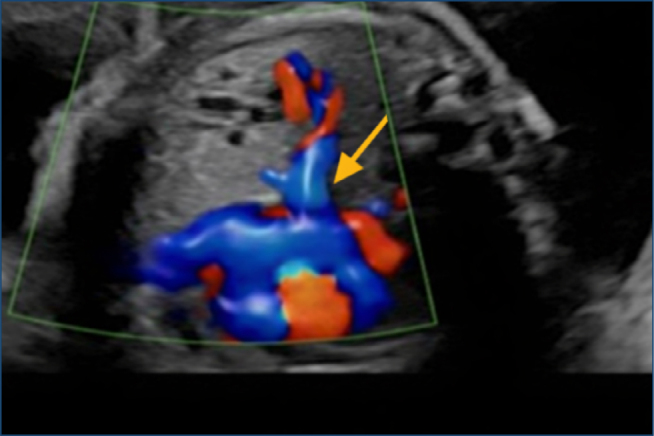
Color Doppler imaging demonstrates pulmonary arterial branching within the lesion.
